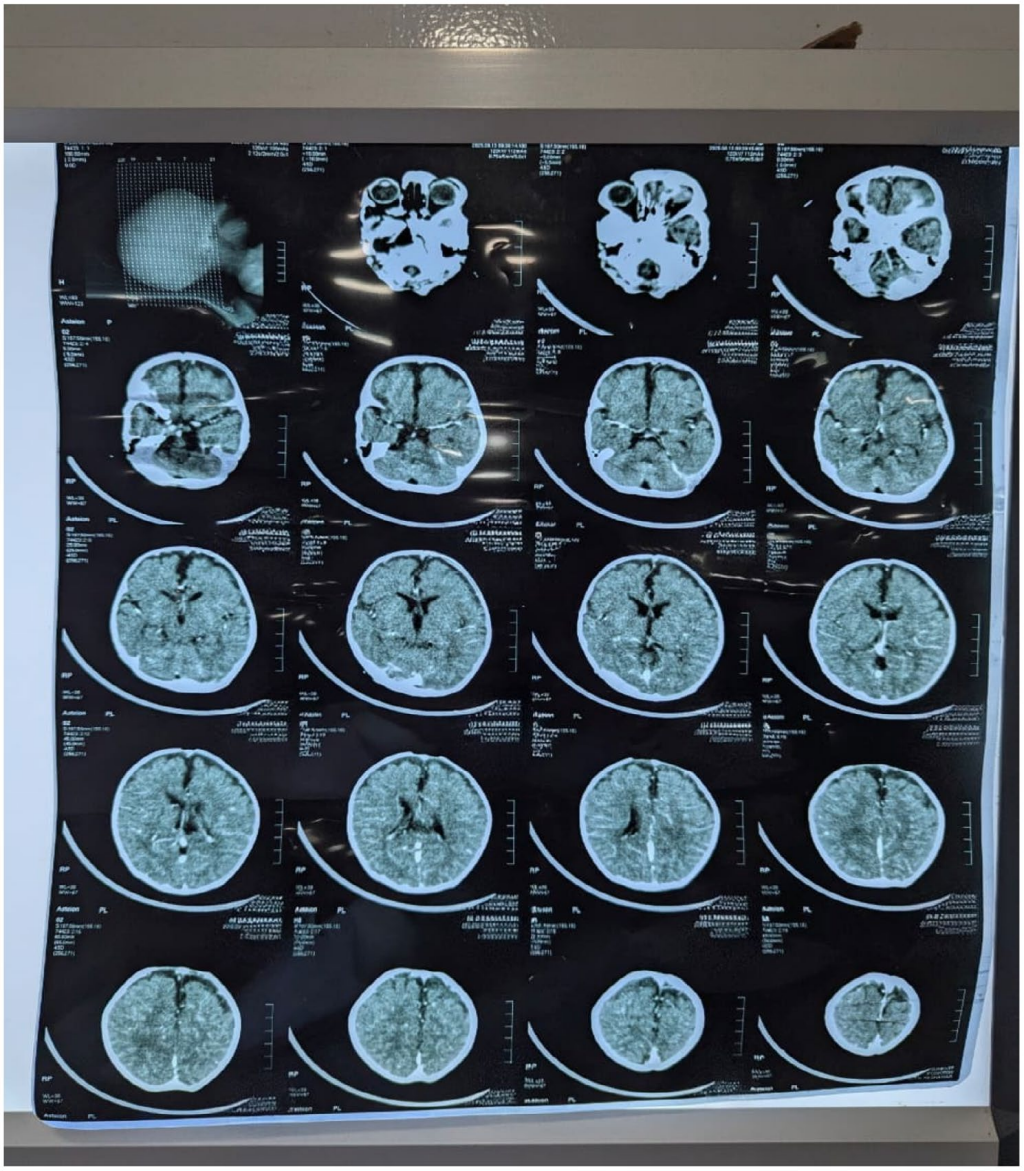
CT scan of the brain showing diffuse leptomeningeal enhancement and mild bifronto‐temporal subarachnoid space prominence (BESS pattern); no focal lesion or mass effect.
